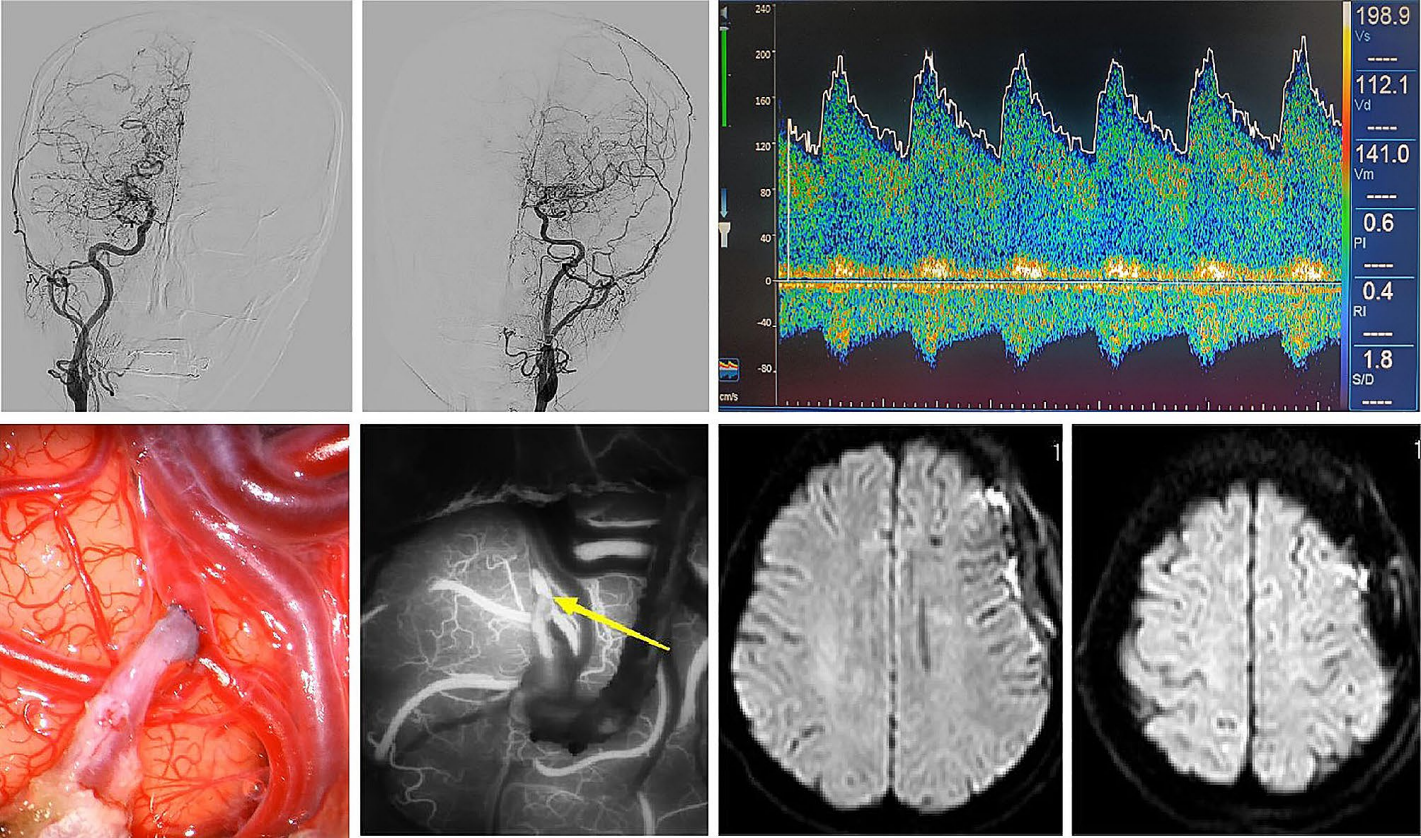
Typical case 1. This a 40-year-old female patient, who was treated for half a year because of headache and weakness of the right limb. After admission, the patient was diagnosed as moyamoya disease by DSA examination, Suzuki stage III. TCD examination showed that the PSV, EDV and MFV of the left MCA were 198.9 cm/s, 112.1 cm/s and 141.0 cm/s. The PI was 0.615. Then the patient underwent left STA-MCA vascular anastomosis combined with EDMS. During the operation, the anastomotic stoma was full and good, and ICG fluorescein angiography showed that the bridging vessel was unobstructed. No new cerebral infarction was found in MRI after operation. The patient recovered well after operation and was discharged without complications related to neurological function
